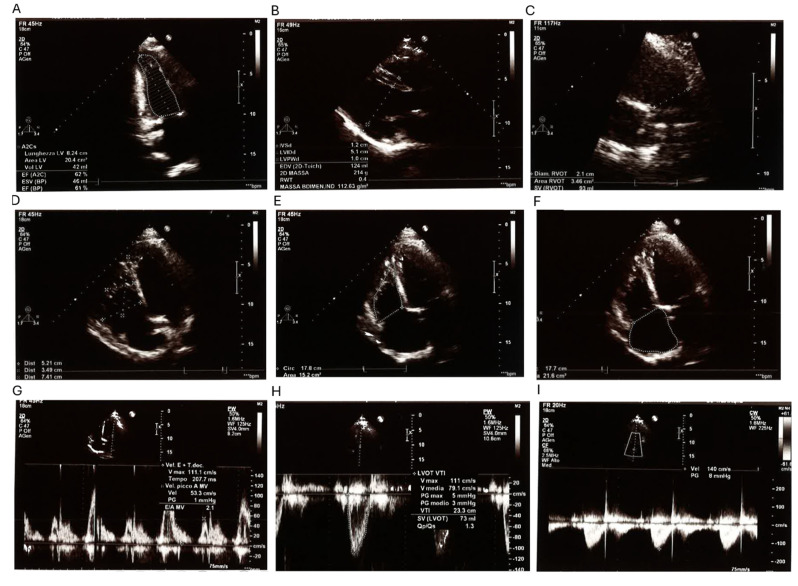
Comprehensive transthoracic echocardiographic assessment in a patient with a history of perimembranous ventricular septal defect (VSD) repair, patent foramen ovale (PFO) closure, and pulmonary artery plasty. (A) Apical two-chamber view showing a left ventricular (LV) end-diastolic volume of 42 mL, end-systolic volume of 46 mL, and preserved systolic function with ejection fraction (EF) of 62%. (B) Parasternal long axis view measuring interventricular septum (IVSd: 1.2 cm), LV internal diameter (LVIDd: 5.1 cm), posterior wall (LVPWd: 1.0 cm), and LV mass (214 g; indexed LV mass: 112.63 g/m^2^). (C) Right ventricular outflow tract (RVOT) view, with diameter of 2.1 cm, cross-sectional area of 3.46 cm^2^, and stroke volume of 93 mL. (D) Apical view of right chambers showing mild right atrial dilation (TD area: 30 cm^2^). (E) Four-chamber view demonstrating mild left atrial enlargement (area: 15.2 cm^2^) and eccentric tricuspid regurgitation directed toward the atrial septum. (F) Left atrial volume estimation (21.6 cm^3^). (G) Pulsed-wave Doppler of mitral inflow showing an E/A ratio of 2.1, suggestive of normal LV filling pressures. (H) LVOT Doppler showing VTI of 23.3 cm, stroke volume of 73 mL, and Qp/Qs ratio of 1.3, indicating a small residual left-to-right shunt. (I) Continuous-wave Doppler showing a pulmonary peak velocity of 140 cm/s and a pressure gradient of 8 mmHg, consistent with mild residual pulmonary valve insufficiency. Overall, systolic function is preserved, and there are no signs of pericardial effusion. Right-sided sections are mildly dilated with preserved contractility (TAPSE 25 mm, FAC 47%). The inferior vena cava is not dilated and shows normal collapsibility.
